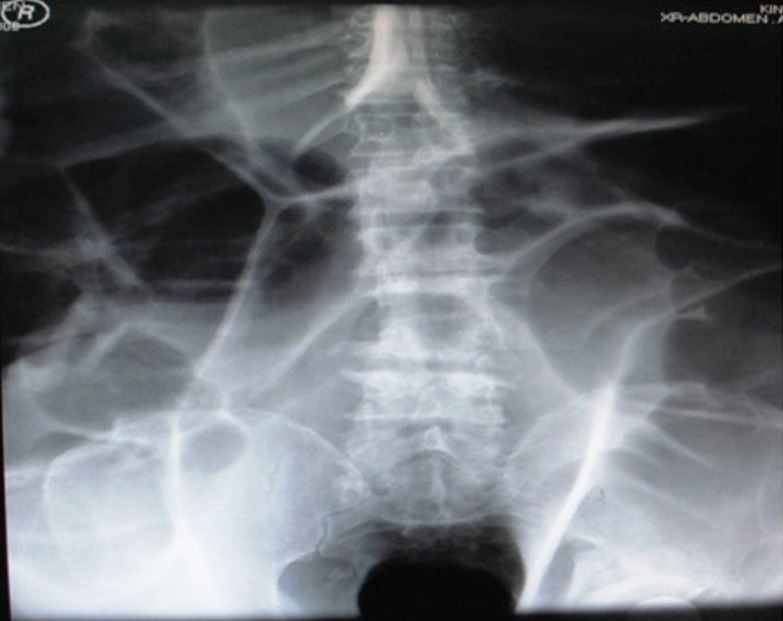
Portable plain abdomen X-ray showing grossly hugely dilated colon with bowel wall edema. The lateral walls are not seen due to huge distension
